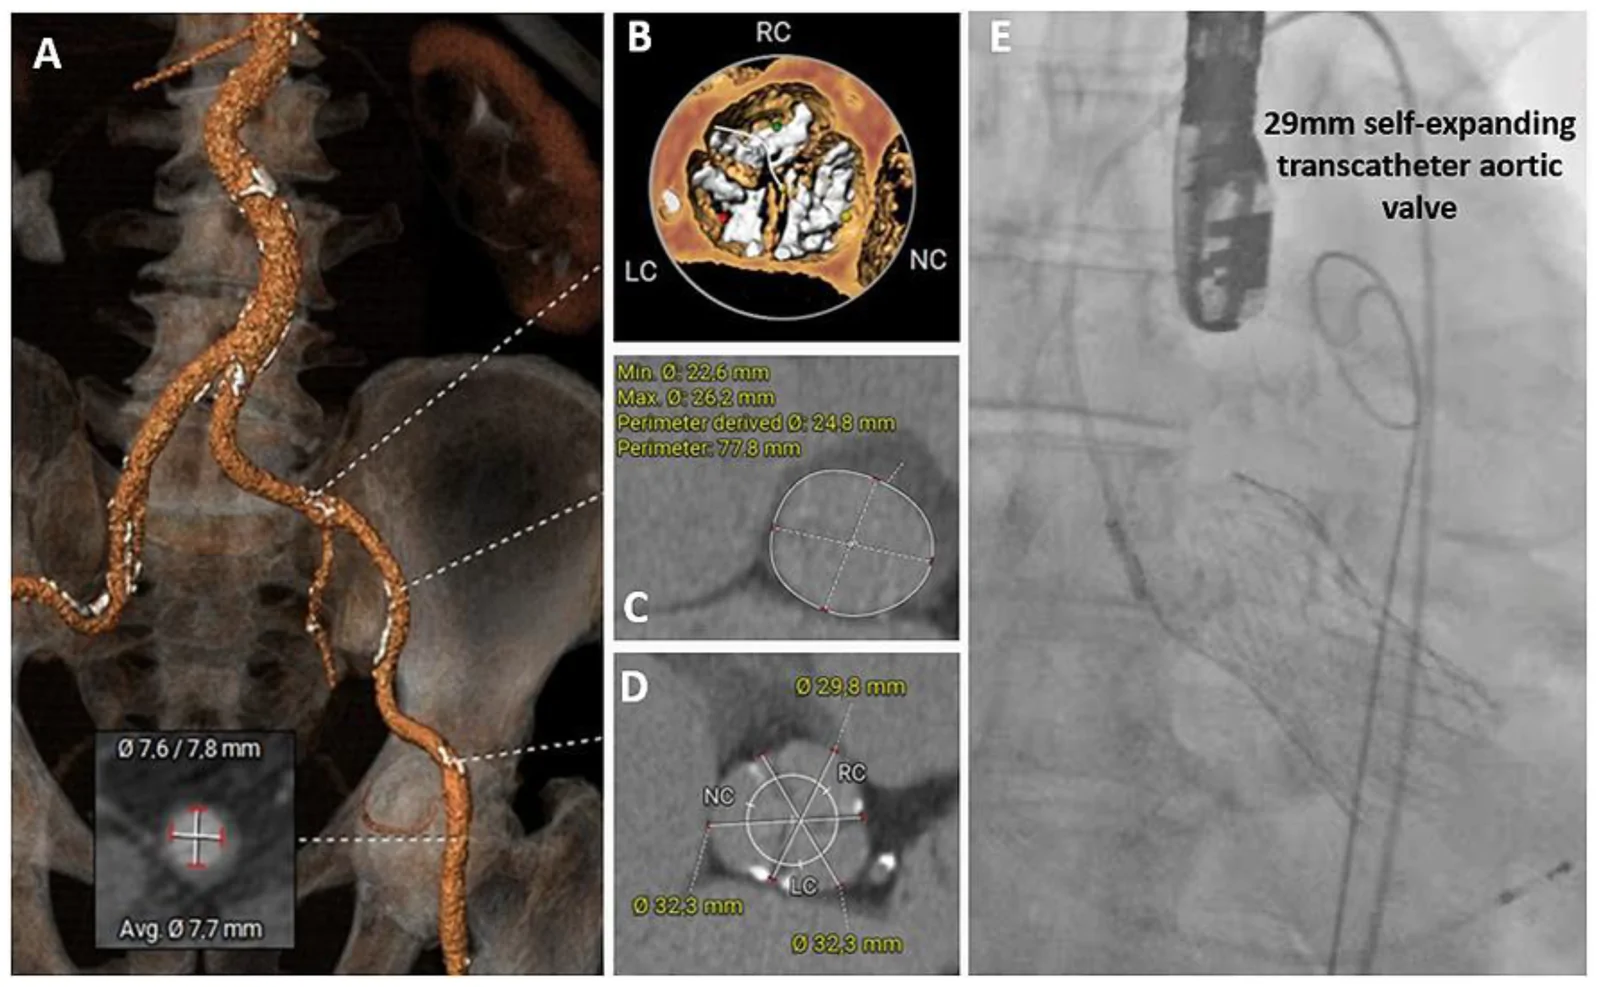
Pre-procedural multidetector CT and procedural imaging assessment for TAVI planning and implantation. (A) Favorable iliofemoral access; (B) Tricuspid, heavily calcified aortic valve; (C) Aortic annulus with a mean diameter of 24.4 mm, perimeter of 77.8 mm, and area of 476.2 mm^2^; (D) Favorable aortic root anatomy; (E) Successful implantation of a 29-mm self-expanding transcatheter aortic valve under combined fluoroscopic and TOE guidance.
